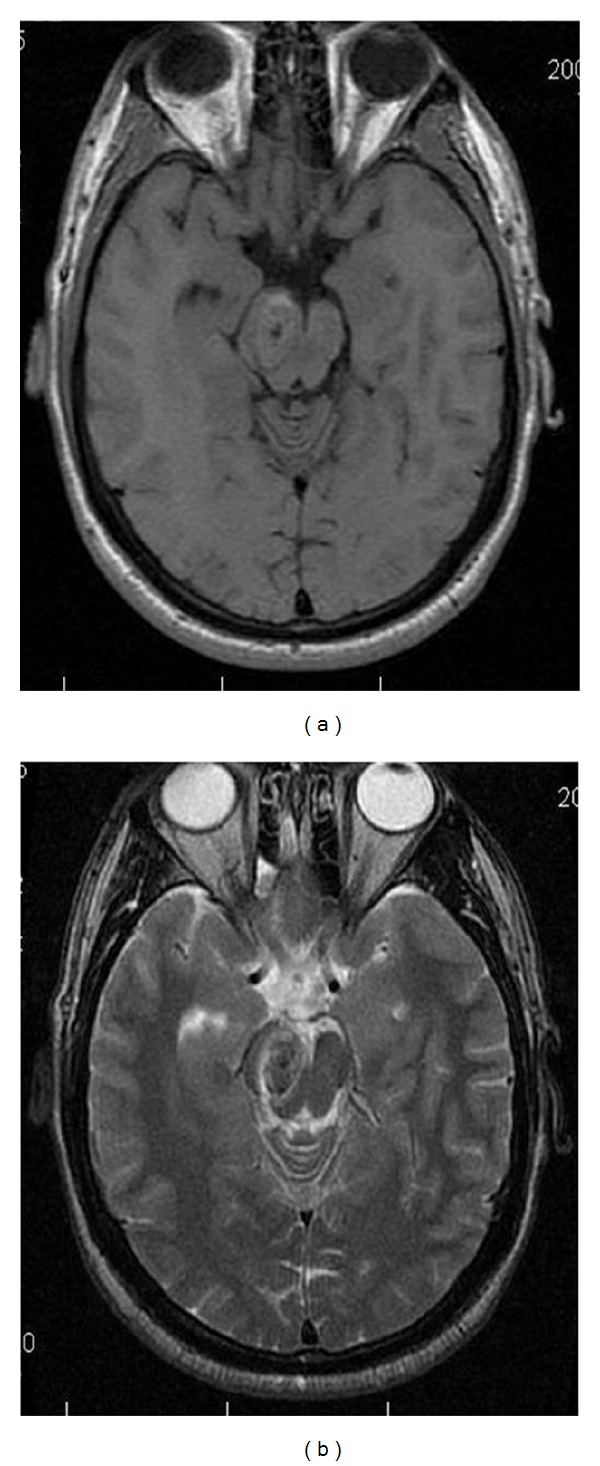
Benedikt Syndome. Ovoid lesion within the midbrain demonstrates isointensity on axial T1-weighted image (a), and slight hypointensity on axial T2-weighted (b) image. These findings are consistent with a cavernous hemangioma within the right midbrain, presenting with symptoms of Benedikt Syndrome.
